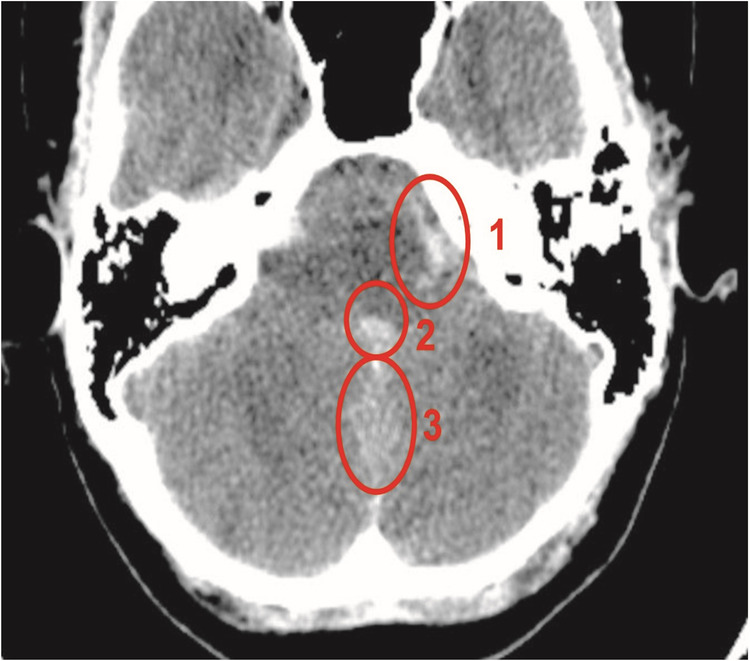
A non-contrast CT head without contrast demonstrating spontaneous subarachnoid haemorrhage (SAH) in the basal cisterns (circle 1), in the fourth ventricle (circle 2), and along the cerebellar hemispheres (circle 3) caused by ruptured left posterior inferior cerebellar artery (PICA) aneurysm.
